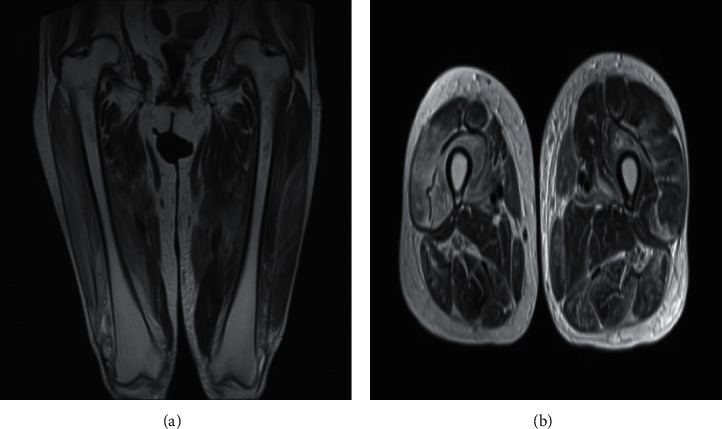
Magnetic resonance imaging (MRI) of bilateral thigh muscles of a woman who developed recurrent anti-glycyl tRNA synthetase (EJ) antibody-positive dermatomyositis concurrently with newly developed nephrotic syndrome shown are sagittal (a) and axial. (b) MRI images demonstrating wide-spread high-intensity lesions on T2-weighted sequences.
